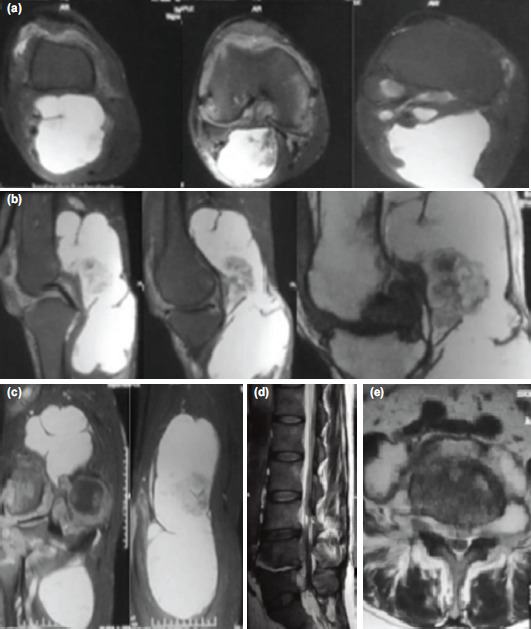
MR images of the knee and lumbosacral spine. (a) Axial cut T2 PDS view of the right knee showing a hyperintense mass located posteriorly popliteal fossa (b) Sagittal cut T2 and T1 images of the right knee showing the same hyperintense mass extending completely in the popliteal fossa with a hypointense lesion in the middle suggestive of clumps of hemosiderin deposition (red arrow). (c) Coronal cut T2 images of the right knee indicative of the same lesion and its continuous extent in the popliteal fossa with a probable frond of synovial tissue in the cyst. (d) Sagittal cut T2 images of the lumbosacral spine showing a typical tuberculous paradiscal lesion at L4-L5 level. (e) Axial cut images of the lumbar spine at L4-L5 level suggestive of cord compression due to tuberculous lesion and associated bony destruction.
